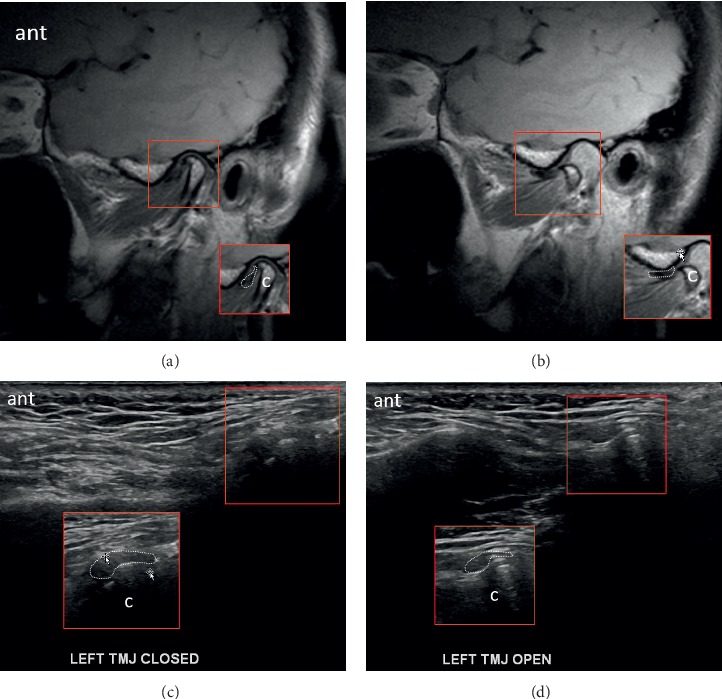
Images demonstrating a deformed disc with fixed anterior dislocation in the sagittal plane in the (a) closed-mouth position with MRI, (b) open-mouth position with MRI, (c) closed-mouth position with US, and (d) open-mouth position with US. A focal annotated view was provided to aid visualization with the articular disc outlined with a dotted contour; condyle = “c”; anterior = “ant”; left = “LT.”
